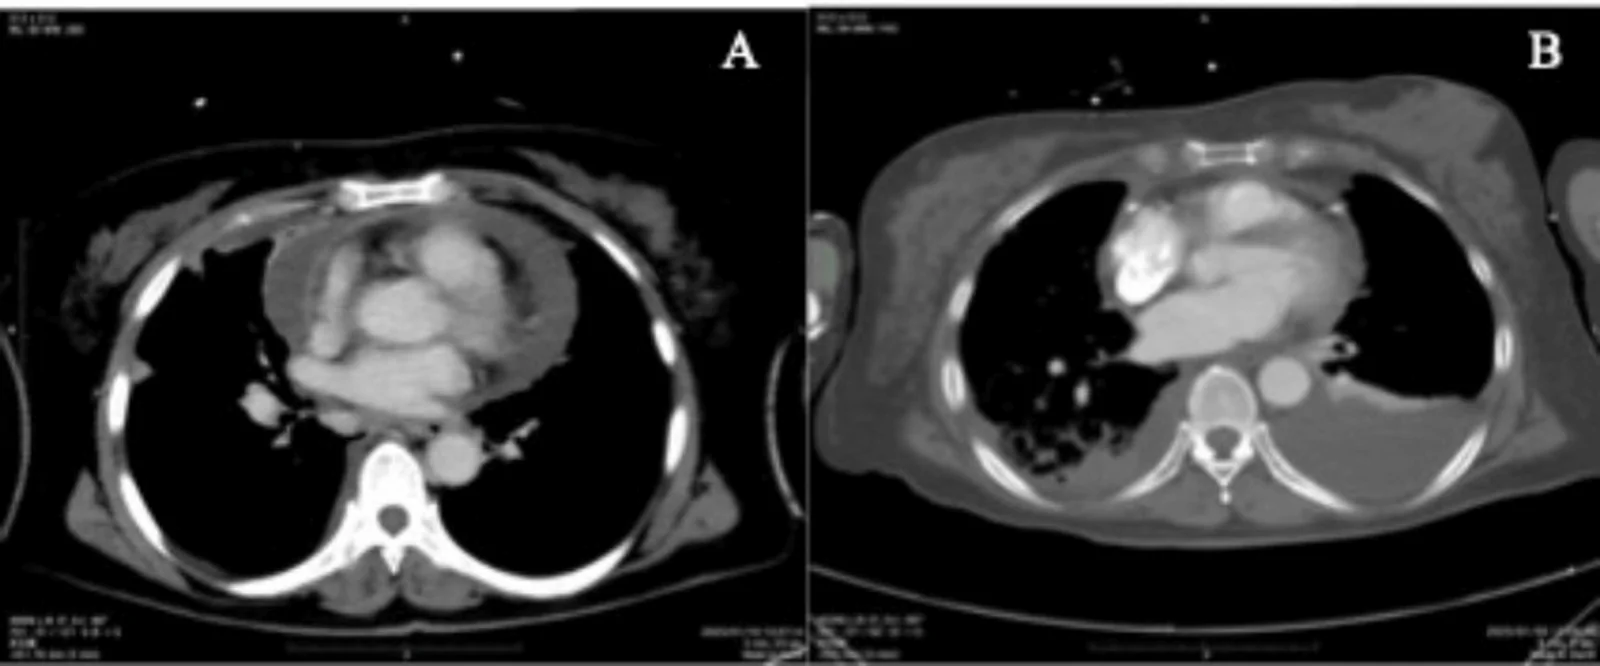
CT scan, on admission and on day 12 of hospitalization Pericardial effusion was noted on admission (A), but the volume decreased after drainage via pericardiocentesis (B). However, progressive diastolic dysfunction was observed, raising suspicion of constrictive pericarditis. Nevertheless, no obvious pericardial calcification, significant pericardial thickening (pericardium 4 mm), or signs of pericardial inflammation were observed (B).
